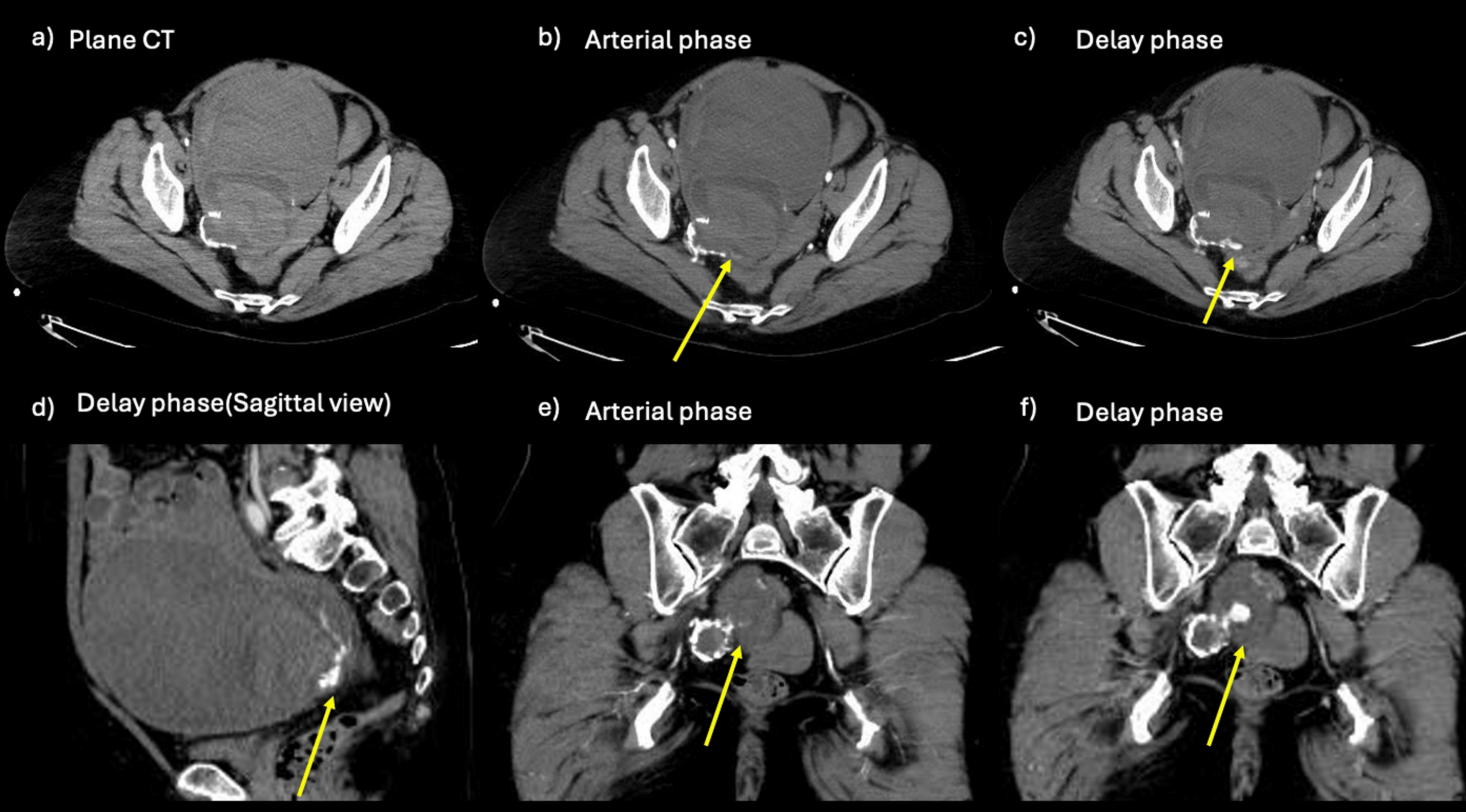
CT images at the previous hospital Although the imaging findings were almost the same as in Figure 2, the extravascular leakage was minute compared to Figure 2. The patient was transferred because the bleeding source was misidentified as ovarian, and hemostasis with transcatheter arterial embolization (TAE) was considered difficult. (a) A well-defined mass lesion with calcification is seen. An even larger structure is visible ventral to the mass lesion (findings unchanged from Figure 2a). (b, e) Contrast-enhanced CT (arterial phase) shows that one part of the lesion lacks calcification. Slight extravascular leakage (arrow) is seen external to the calcified area (unlike Figure 2c, this shows considerably less extravascular leakage). (c, d, f) Contrast-enhanced CT (delayed phase) shows that the extravascular leakage has enlarged (arrow).
